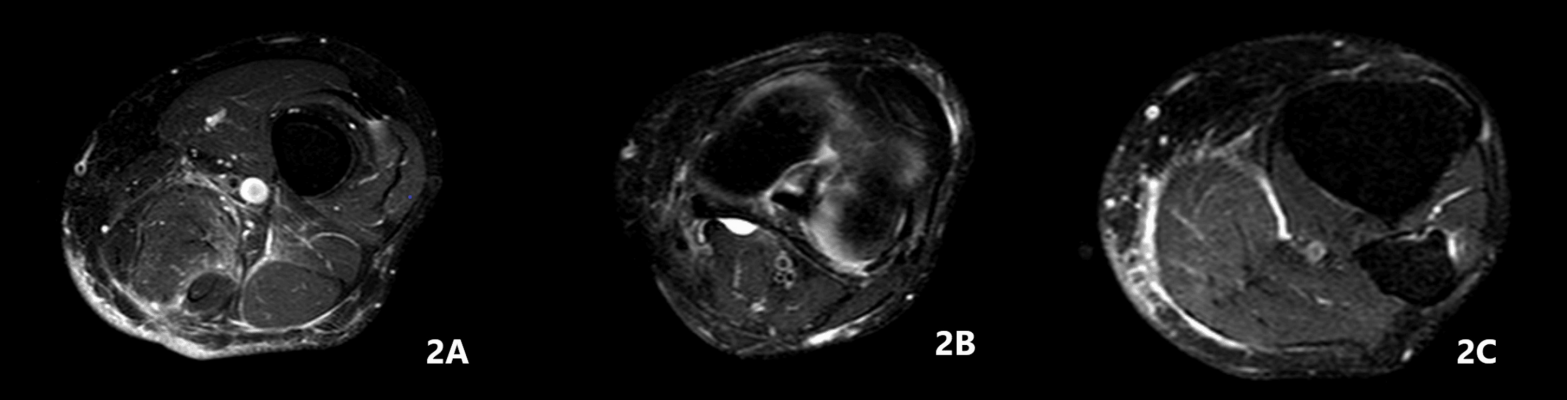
Postoperative MRI scan. A. No cyst observed in the semimembranosus muscle. B. Baker cyst, smaller than in the preoperative CT scan. C. No cyst observed in the proximal gastrocnemius muscle.
